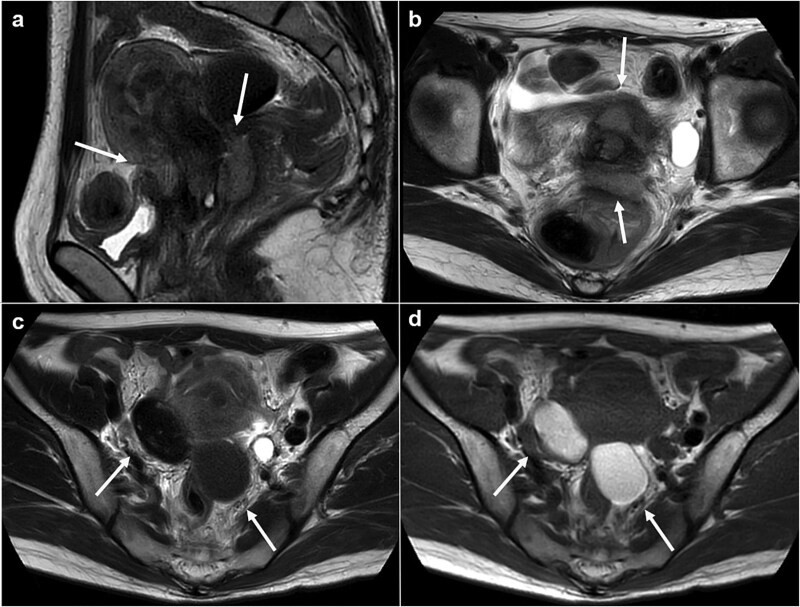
Magnetic resonance images. (a) Sagittal T2 weighted image. (b) Axial T2 weighted image. (c) Axial T1 weighed image. (d) Axial T2 weighed image. Tumors are observed at either the anterior or posterior portion of the urine cervix (a, b: arrows). Ovarian endometriosis is observed in both ovaries (c, d: arrows). Walls of the cysts are smooth.
